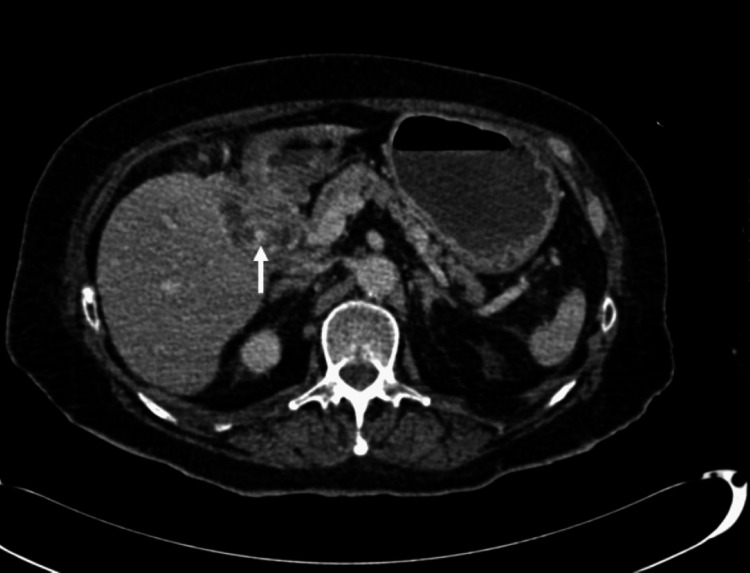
Axial portal venous CT shows gallstone fistulated into the duodenum (white arrow) with gastric dilatation.
